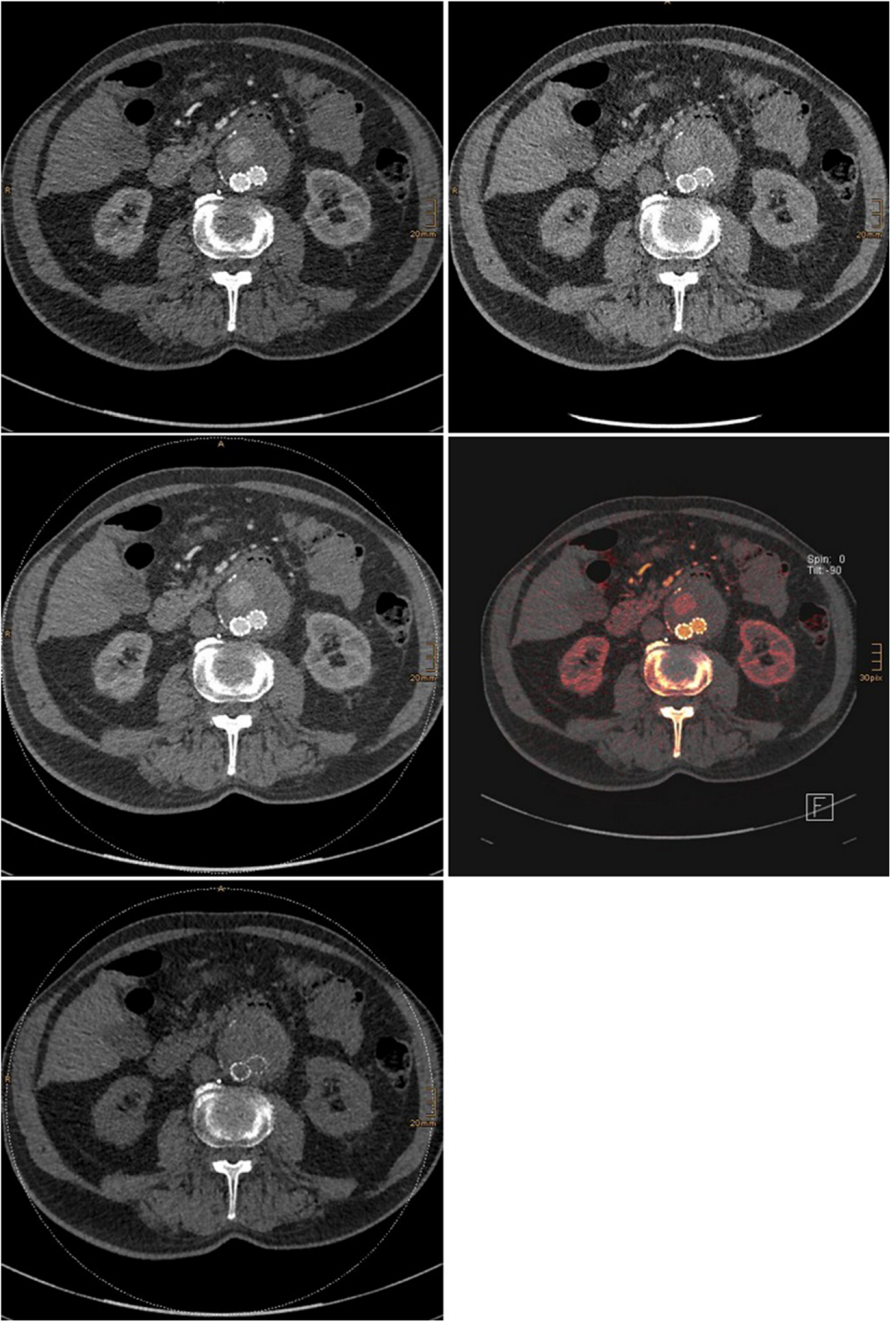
The first three images show the 80-kVp, 140-kVp, and mixed or fused images. Iodine colour-coded (fourth image) and virtual non-contrast (fifth image) CT data sets are also shown
